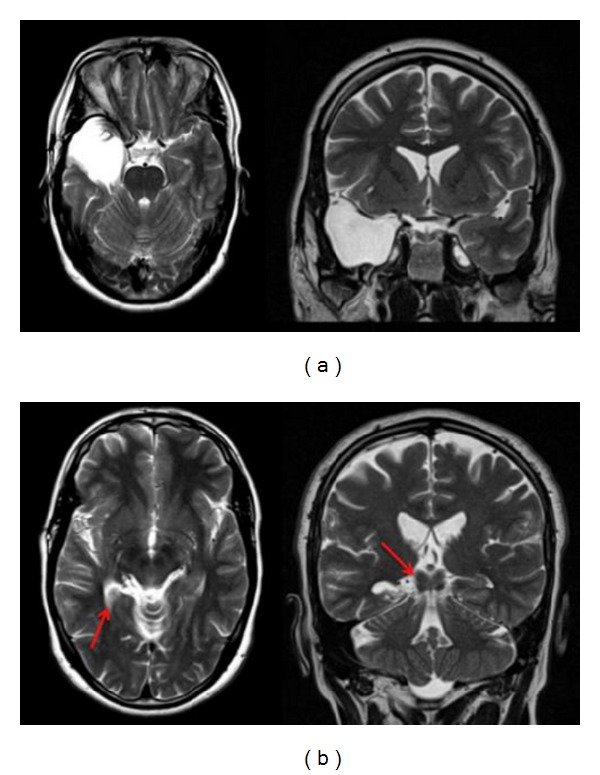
Postoperative MRI brain (axial and coronal T2 weighted images) showing the extent of neocortex (a) and posterior hippocampus resection (b). Note: the posterior resection beyond the level of brainstem folliculi (quadrigeminal plate level) (see red arrow).
